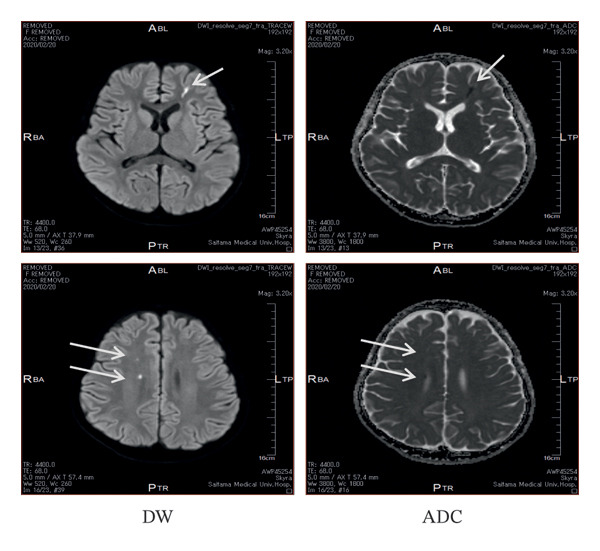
Pretreatment brain magnetic resonance imaging examination revealed high signal intensity areas in the bilateral white matter of the frontal lobe on diffusion‐weighted imaging and low signal intensity areas in the same region on the apparent diffusion coefficient map.
